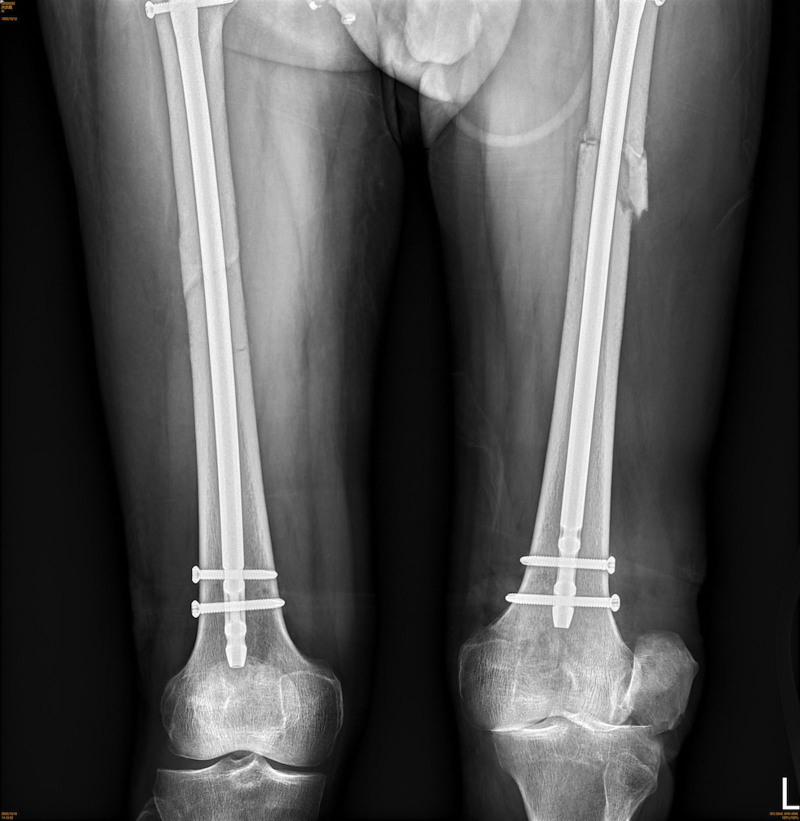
The X-ray image shows patellar dislocation following open reduction of the pelvic and bilateral femoral fractures caused by boat-propellers.
